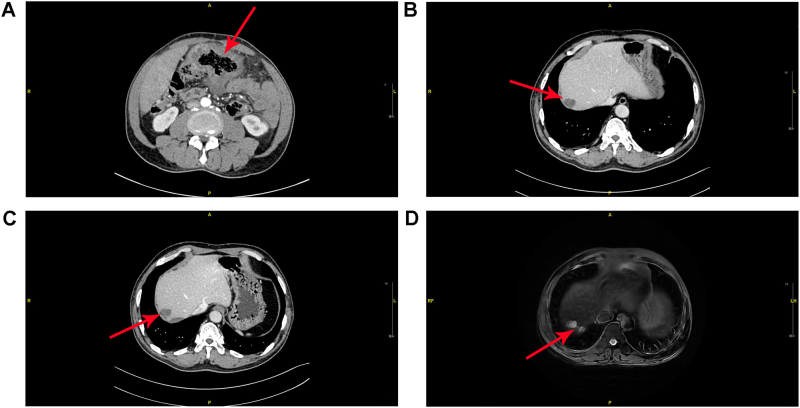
Computed tomography (CT) scan of the abdomen performed on admission and MR images of the upper abdomen during the postoperative follow-up. (A) Preoperative CT indicated marked dilatation from the hepatic flexure to the transverse colon with associated bowel wall thickening (red arrow), raising suspicion for colon cancer. (B) In the 10 months follow-up, CT scan indicated a subcapsular low-density lesion (red arrow) in the right hepatic lobe, consistent with suspected hepatic metastasis. (C) After sintilimab-based immunotherapy, the patient showed no significant progression (red arrow) at 22 months compared with the 10-month follow-up. (D) On T2-weighted imaging, the metastatic lesion in the right hepatic lobe demonstrates mildly increased signal intensity with a slight interval increase in size (red arrow), as revealed on upper abdominal MR.
